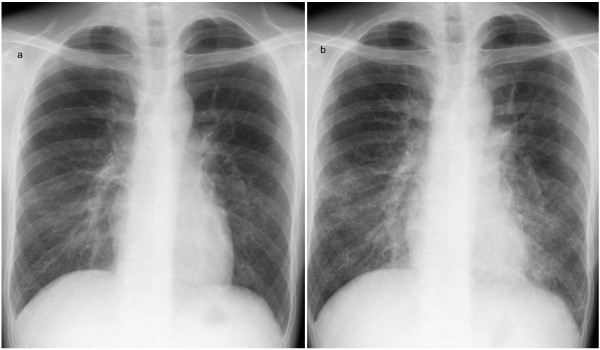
Chest radiographs taken at our patient’s first (a) and second (b) admission. The chest radiograph from the second admission (b) showed a bilateral interstitial pattern.
